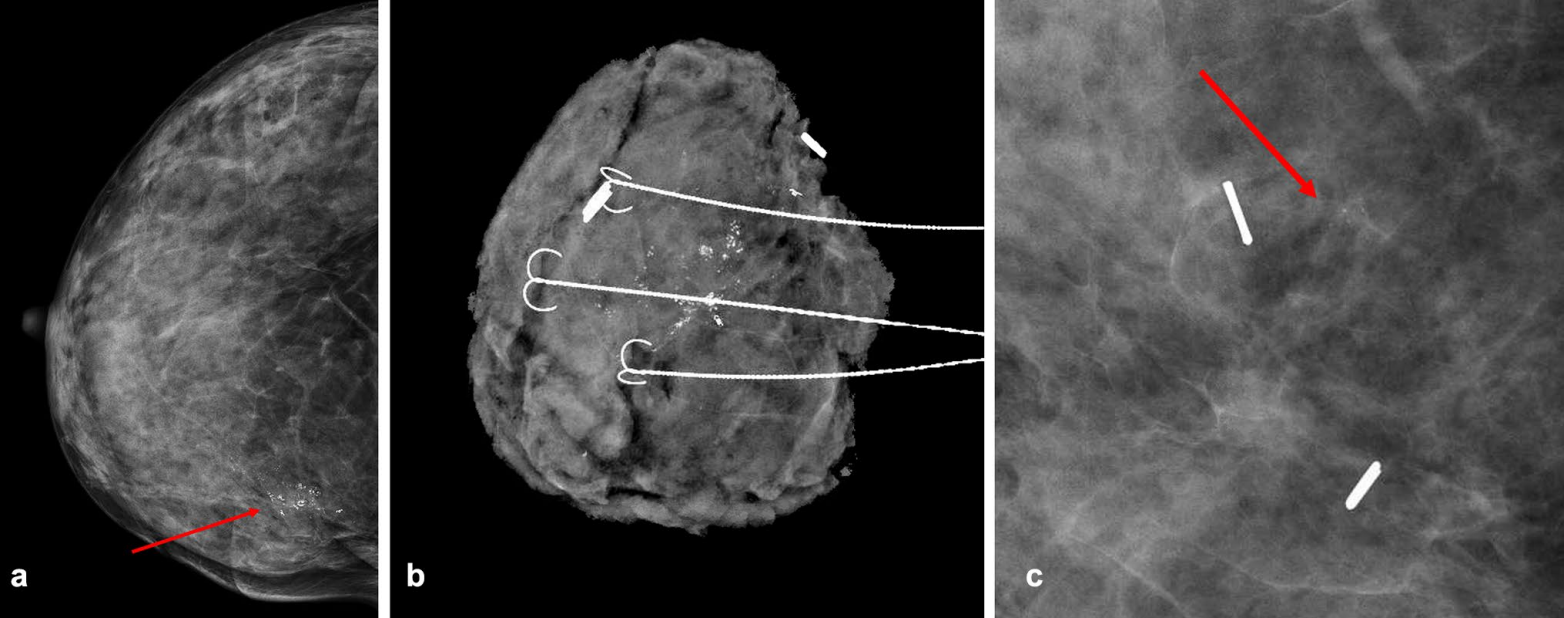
A Pre-operative mammogram, CC view, showing a large cluster of suspicious calcifications at the inner part of the right breast. Mammotome biopsy showed DCIS (arrow). B Intra-operative X-ray showing excised calcifications. C Post-operative ammogram showing residual malignant calcifications in the surgical bed (arrow)
